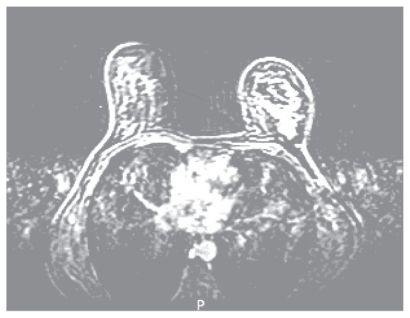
Baseline dynamic contrast enhanced breast MRI demonstrating extensive malignant type enhancement in the left upper outer breast.
